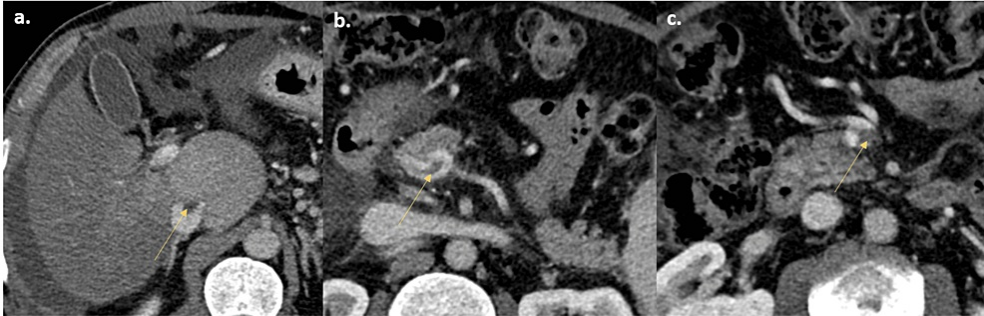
Three sections of abdominal CT with contrast in the venous phase showing venous thrombosis. Venous thrombi are visualized in image (a) in the inferior vena cava (arrow), in image (b) in the splenoportal venous confluence (arrow), and in image (c) in the superior mesenteric vein (arrow).
